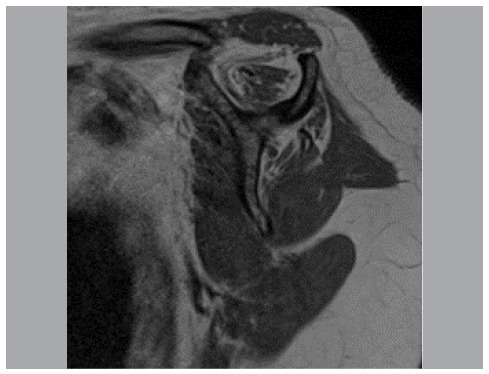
Sagittal section used to assess T1-weighted fatty infiltration.
